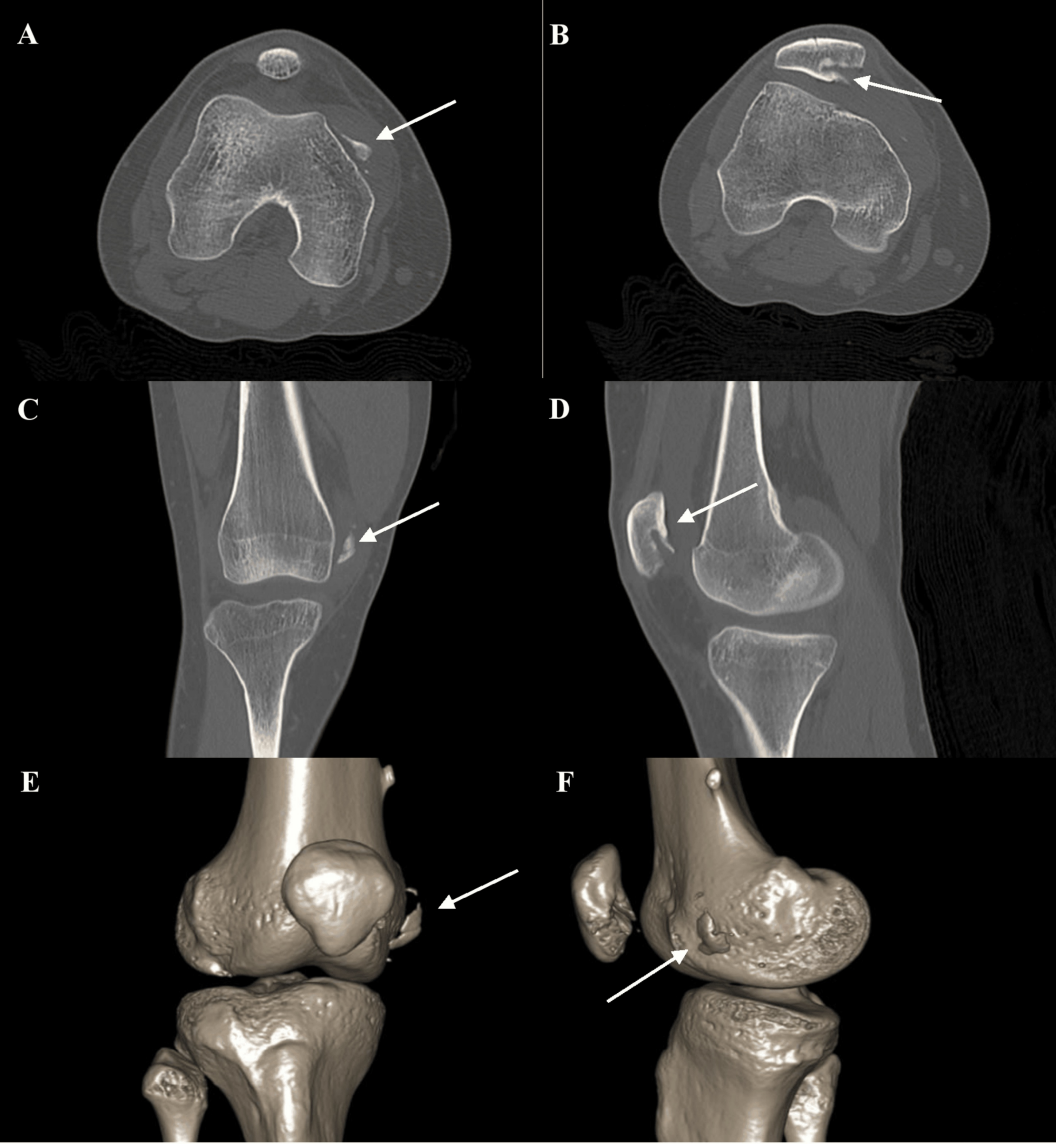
Pre-operative axial (A, B), sagittal (C, D), and CT-based three-dimensional reconstructions (E, F) suggestive of a large osteochondral fragment in the medial gutter consistent in shape with a defect of the inferior aspect of the medial patellar facet (A, C, E, F), with an osteochondral fragment of the posterior lateral femoral condyle embedded inside the inferior aspect of the medial patellar facet (B, D). A: Pre-operative axial CT image suggestive of large osteochondral fragment in medial gutter consistent in shape to defect of inferior aspect of medial patellar facet. B: Pre-operative axial CT image suggestive of osteochondral fragment of posterior lateral femoral condyle embedded inside of the inferior aspect of the medial patellar facet. C: Pre-operative sagittal CT image suggestive of large osteochondral fragment in medial gutter consistent in shape to defect of inferior aspect of medial patellar facet. D: Pre-operative sagittal CT image suggestive of osteochondral fragment of posterior lateral femoral condyle embedded inside of the inferior aspect of the medial patellar facet. E: Pre-operative CT based three-dimensional bone reconstruction of lateral gutter suggestive of large osteochondral fragment in medial gutter consistent in shape to defect of inferior aspect of medial patellar facet. F: Pre-operative CT based three-dimensional bone reconstruction of medial gutter suggestive of large osteochondral fragment in medial gutter consistent in shape to defect of inferior aspect of medial patellar facet. White arrows in A,C,E,F osteochondral fragment consistent in shape to defect of inferior aspect of medial patellar facet and those in B and D osteochondral fragment of posterior lateral femoral condyle embedded inside of the inferior aspect of the medial patellar facet. Images reconstructed at Penn State 3D Reconstruction Lab.
